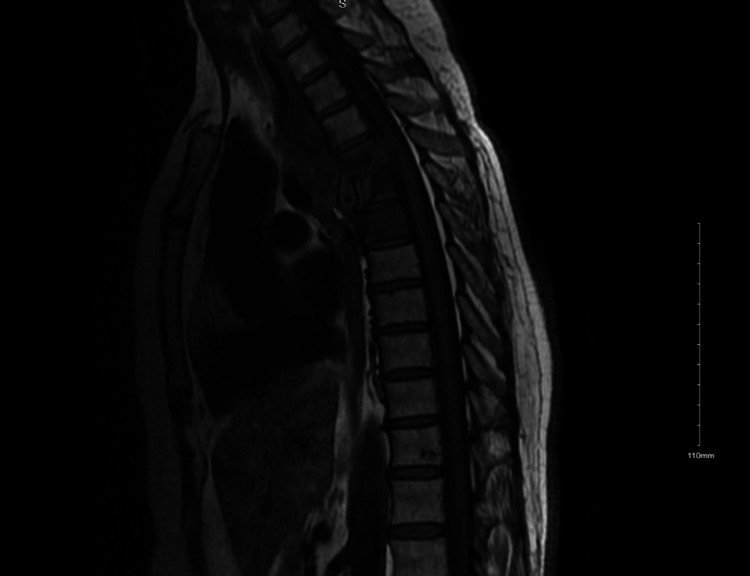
MRI of the thoracic spine
